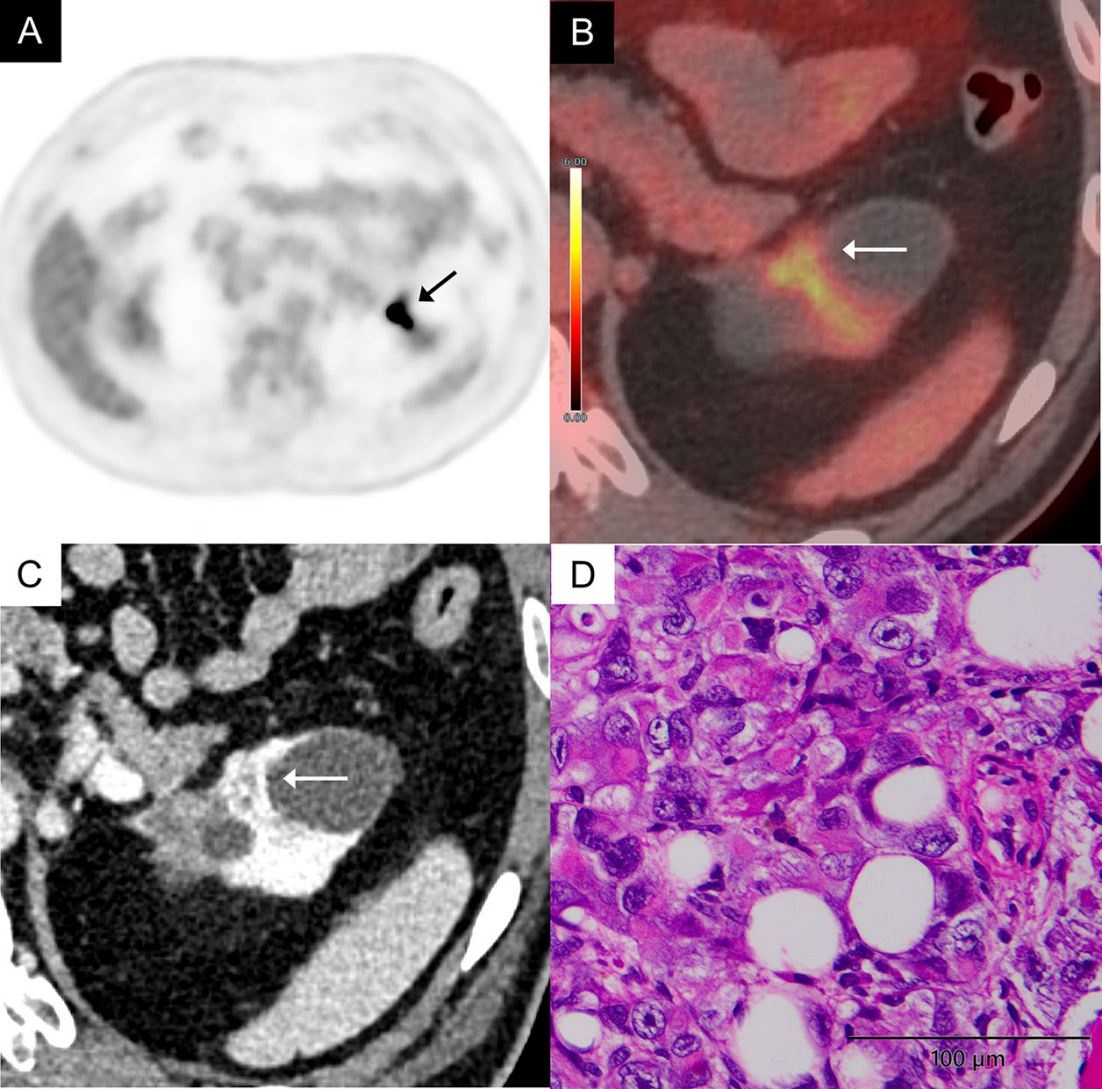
A 54-year-old man with a fumarate hydratase-deficient renal cell carcinoma of the left kidney. The patient had a history of hereditary leiomyomatosis and renal cell cancer syndrome. A Axial FDG-PET image shows moderate mass-like FDG uptake in the left kidney. B Axial FDG-PET/CT image shows moderate FDG uptake in a mass of the left kidney (arrow), showing an SUVmax 7.25, while normal renal cortex shows an SUVmax 2.8. C Axial contrast-enhanced CT in the corticomedullary phase shows an enhancement in the mass (arrow). Multiple osteolytic lesion are detected in the thoracic vertebra, ribs and pelvis (images not shown). D The biopsy of the osteolytic lesion in the thoracic vertebra shows the tumor cells which have distinct nucleoli with eosinophilic cytoplasm
